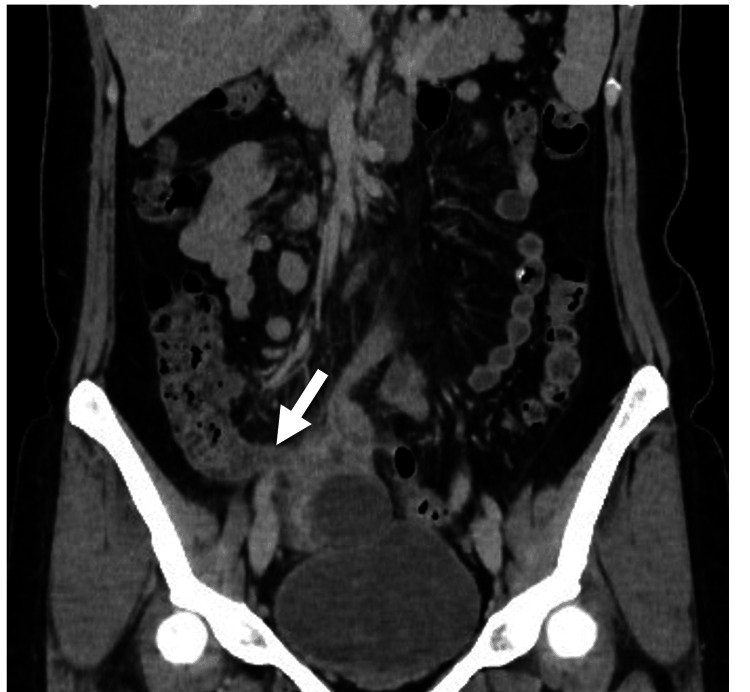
Contrast-enhanced axial abdominal CT revealed localized inflammatory changes in the mesenteric fat adjacent to the ileocecal junction. The appendix was enlarged with wall thickening (arrow).
